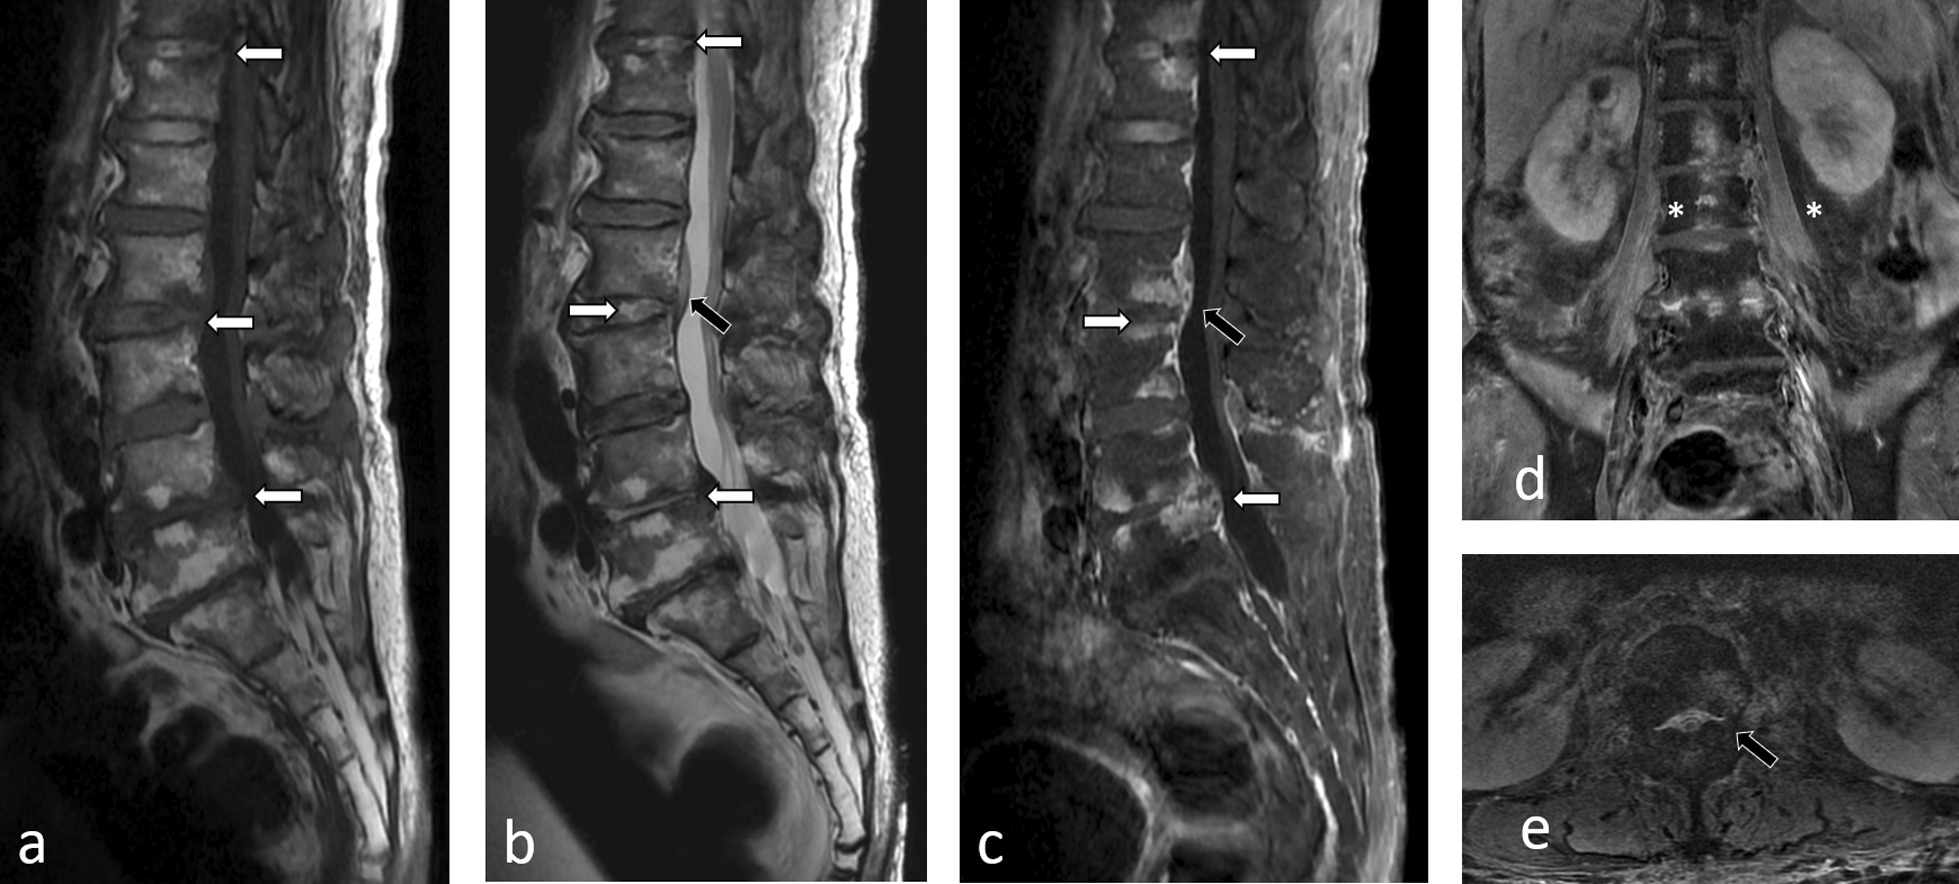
Magnetic resonance (MR) images of the lumbosacral spine. a Sagittal T1-weighted MR image shows endplate irregularity and abnormal low signal intensity (SI) in bone marrow at the T11–T12, L2–L3 and L4–L5 levels (white arrow). b Sagittal T2-weighted MR image shows high SI in the intervertebral disc of T11–T12, L2–L3 and L4–L5 levels (white arrow) and an epidural abscess (black arrow). c Sagittal contrast-enhanced (CE) fat-suppressed T1-weighted MR image shows enhancement of the endplates and bone marrow at the T11–T12, L2–L3 and L4–L5 levels (white arrow) with epidural abscess at L2–L3 level (black arrow). d Coronal CE fat-suppressed T1-weighted MR image shows faint enhancement at bilateral psoas muscles (asterisk) without abscess, represented myositis. e Axial CE fat-suppressed T1-weighted MR image shows an epidural abscess (black arrow)
